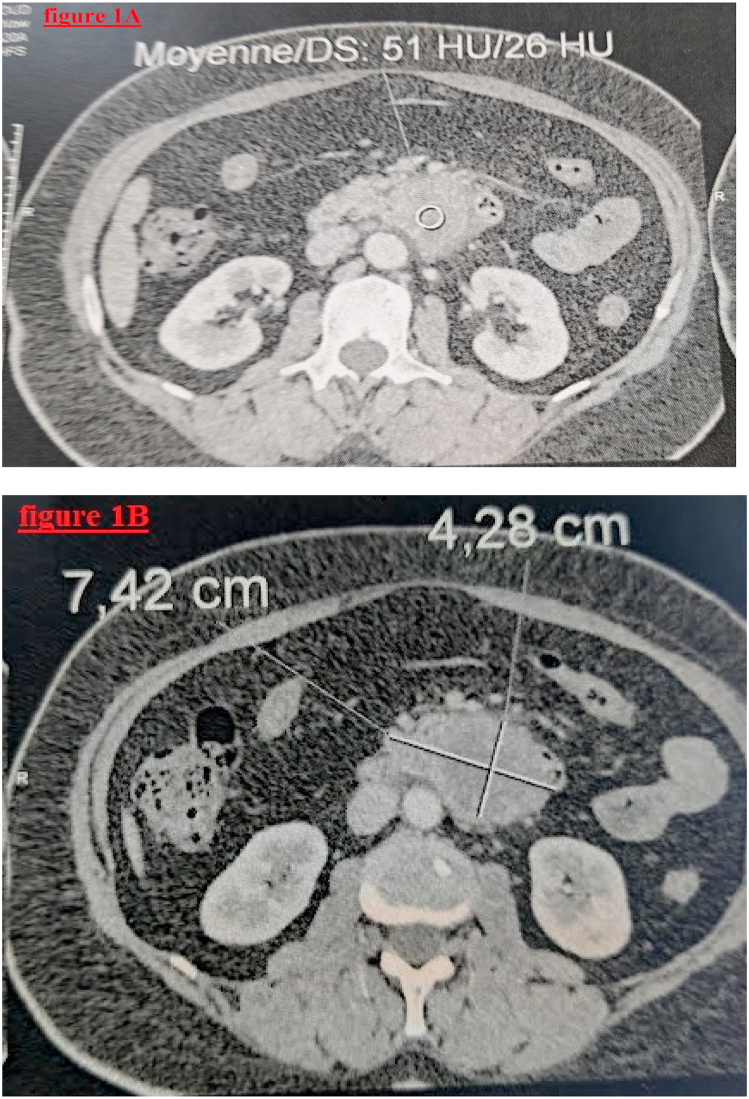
(1A and 1B): MRI shows a retroperitoneal soft tissue tumor, attached to the fourth portion of the duodenum.
